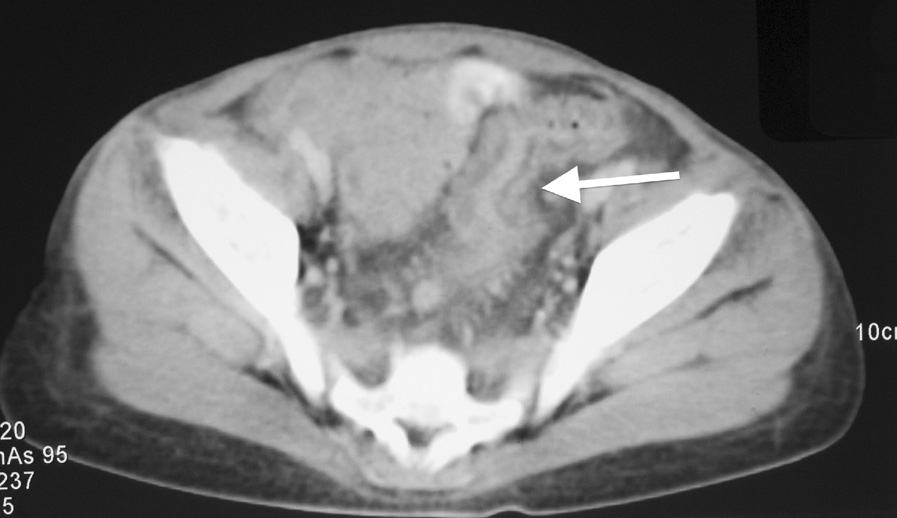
Contrast enhanced computed tomography (CT) of abdomen. There is wall thickening of the sigmoid colon with stranding of adjacent mesenteric fat (arrow). The appearances are of colitis.
